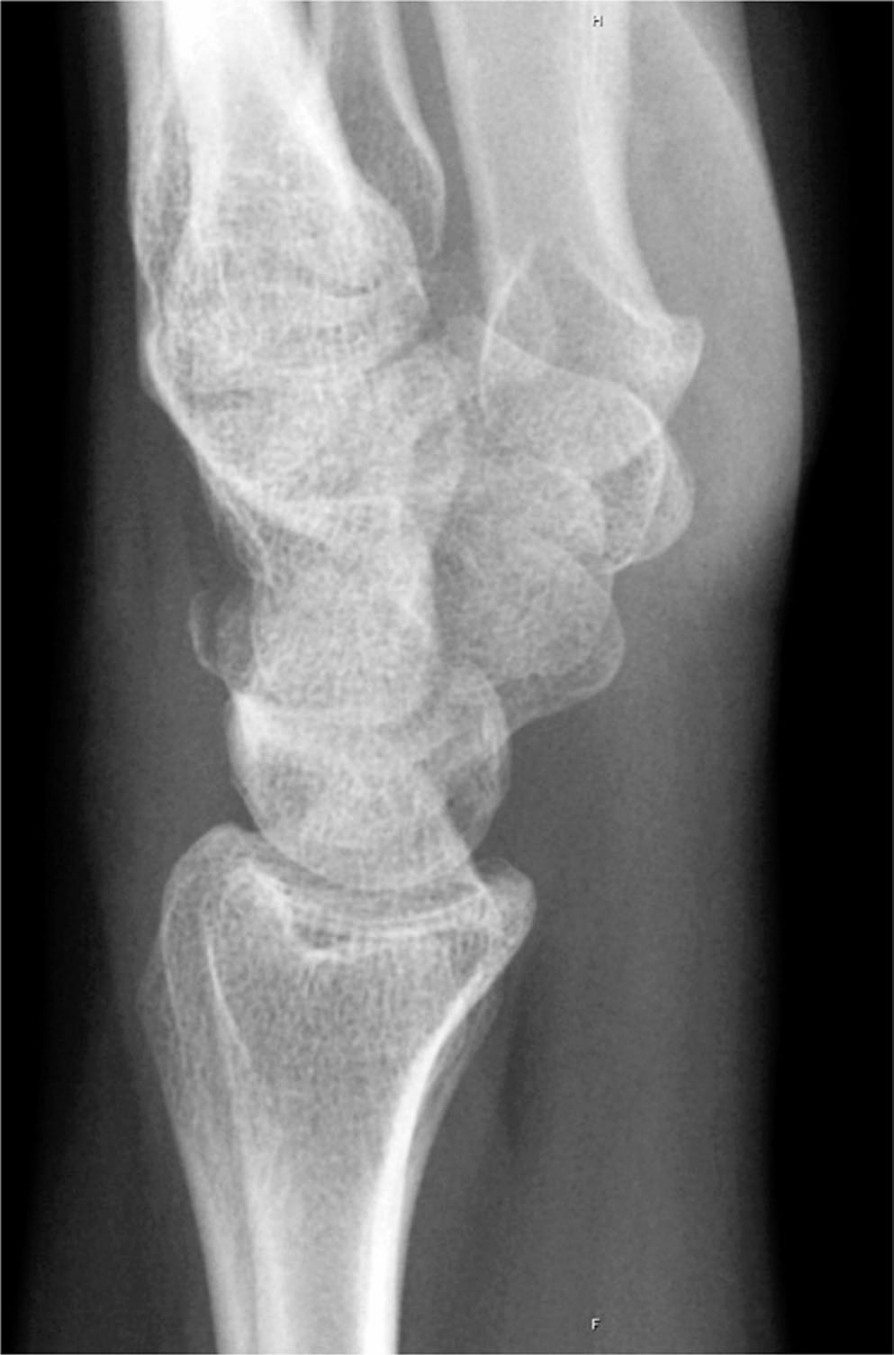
In a lateral wrist x-ray view, a small cortical fragment of the triquetrum is normally seen
